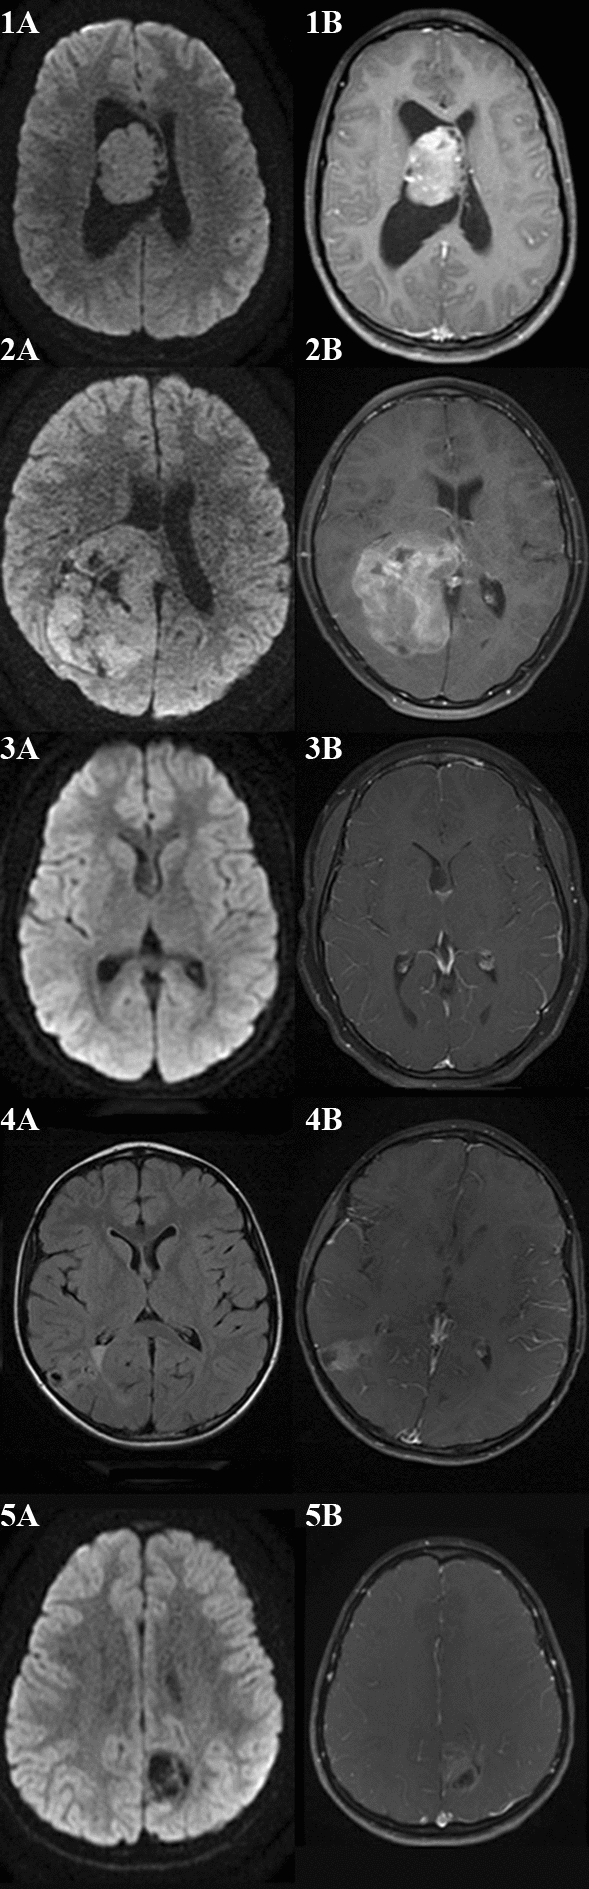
MRI of patient 1 demonstrates a central intraventricular tumor with reduced diffusivity (1A) and avid enhancement on post-gadolinium T1-weighted sequences (1B). MRI of patient 2 demonstrates a large posterior right lateral ventricle tumor with parietal and hemispheric parenchymal spread with reduced diffusivity (2A) and avid heterogenous contrast enhancement on post-gadolinium T1-weighted sequences (2B). MRI of patient 3 demonstrates a subtle intraventricular tumor adjacent to the septum pellucidum and right lateral ventricle on diffusion weighted sequences (3A) with minimal contrast enhancement likely reflecting choroid inferiorly on post-gadolinium T1-weighted sequences (3B). MRI of patient 4 demonstrates a right posterior temporoparietal mixed solid and cystic tumor on T2-weight fluid attenuated inversion recovery sequences (4A) with moderate enhancement on post-gadolinium T1-weighted sequences (4B). MRI of patient 5 demonstrates a mixed solid and cystic left posterior frontoparietal tumor without restricted diffusion on diffusion weighted sequences (5A) and moderate enhancement on post-gadolinium T1-weight sequences (5B).
